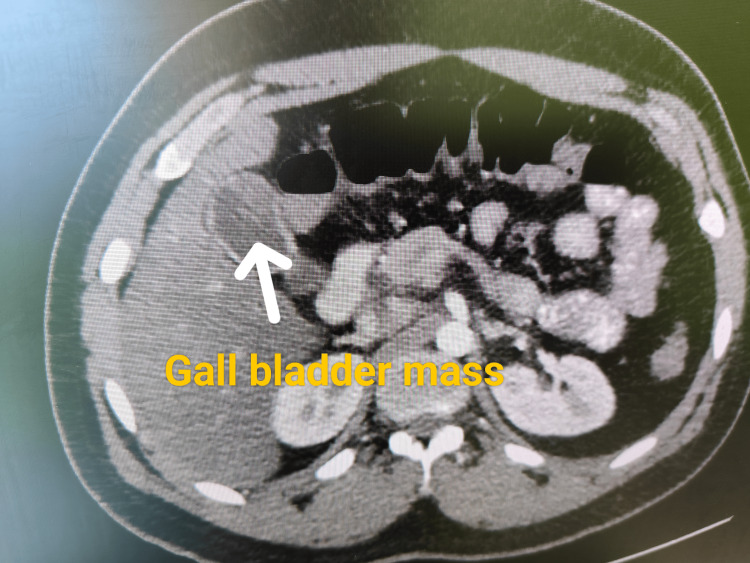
Computed tomography (CT) scan of the abdomen White arrow showing the gallbladder mass
